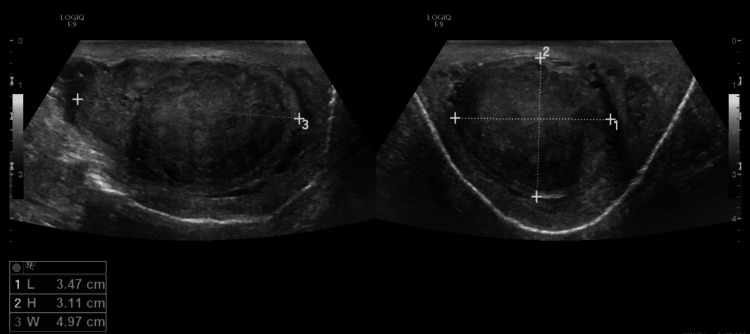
Scrotal ultrasound revealing an intratesticular heterogeneous hypoechoic lesion measuring 3 × 3.6 × 3.4 cm.
